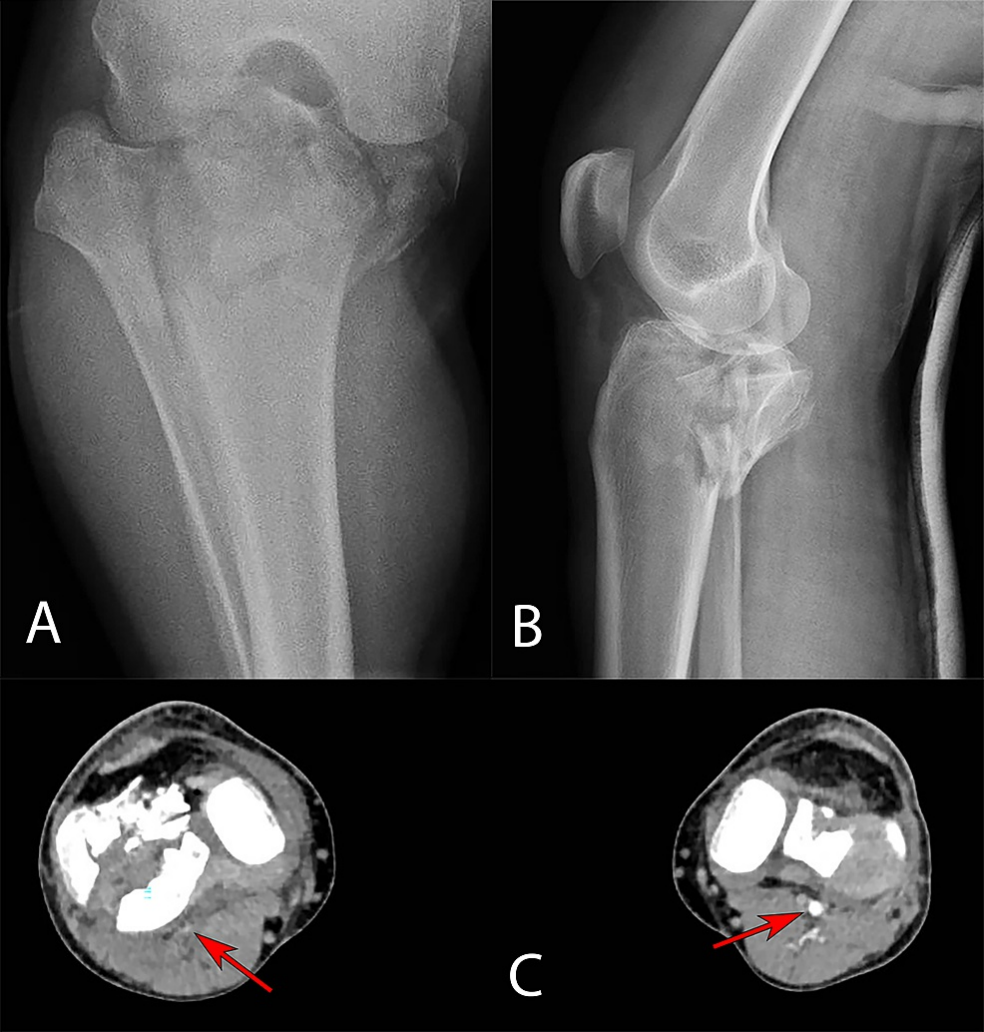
A: Anteroposterior radiograph demonstrating comminuted tibial plateau fracture associated with dislocation. B: Lateral radiograph after realignment was performed demonstrating comminuted tibial plateau fracture. C: Axial cut of computed tomography scan at the level of both tibial plateau demonstrating comminuted right tibial plateau fracture, and near-total obliteration of the right popliteal artery (red arrow). Additionally, note the presence of significant soft tissue swelling of right leg compared to left leg.
